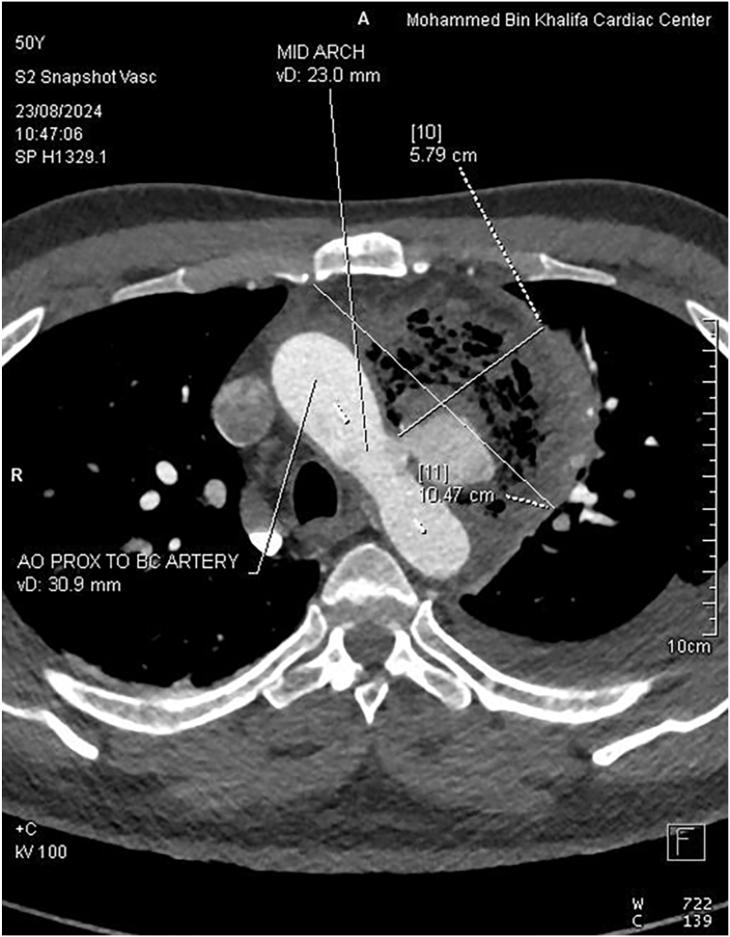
Contrast-enhanced computed tomographic findings. A well-defined lesion in the left anterior mediastinum measured approximately 6.0 cm × 7.0 cm × 8.9 cm and contained both gas and fluid. It was located adjacent to the aortic arch, which showed a pseudoaneurysm. (AO, aorta; BC, brachiocephalic; F, frontal view; PROX, proximate. R, right; +C, contrast-enhanced.)
